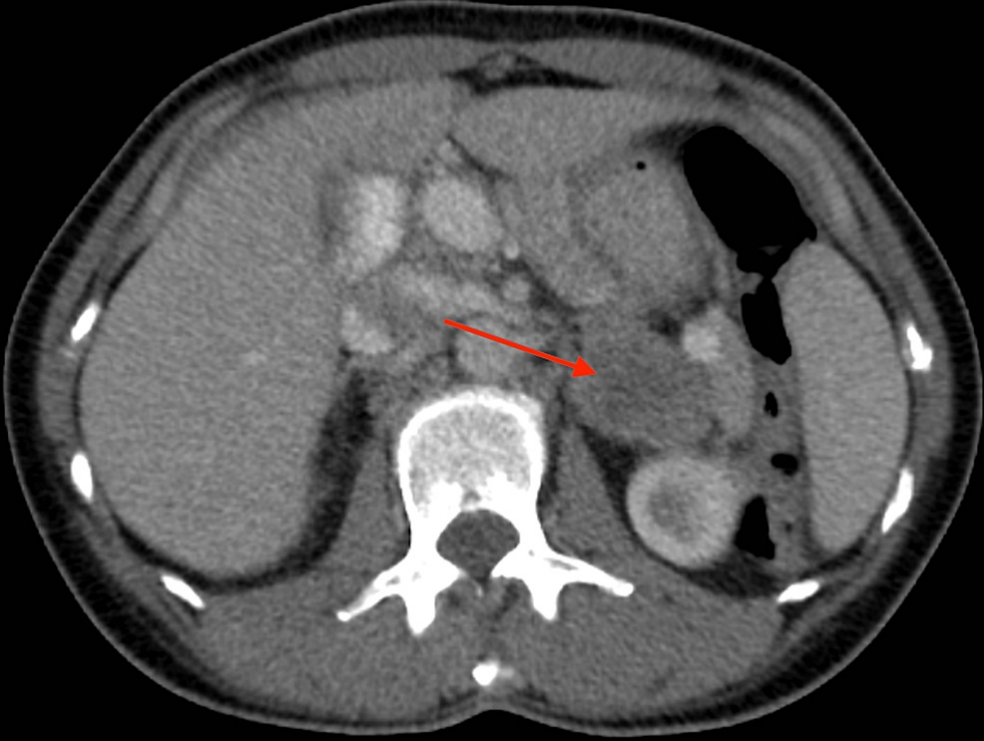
Axial view of non-contrast computed tomography of the abdomen and pelvis demonstrating adrenal mass (arrow).
